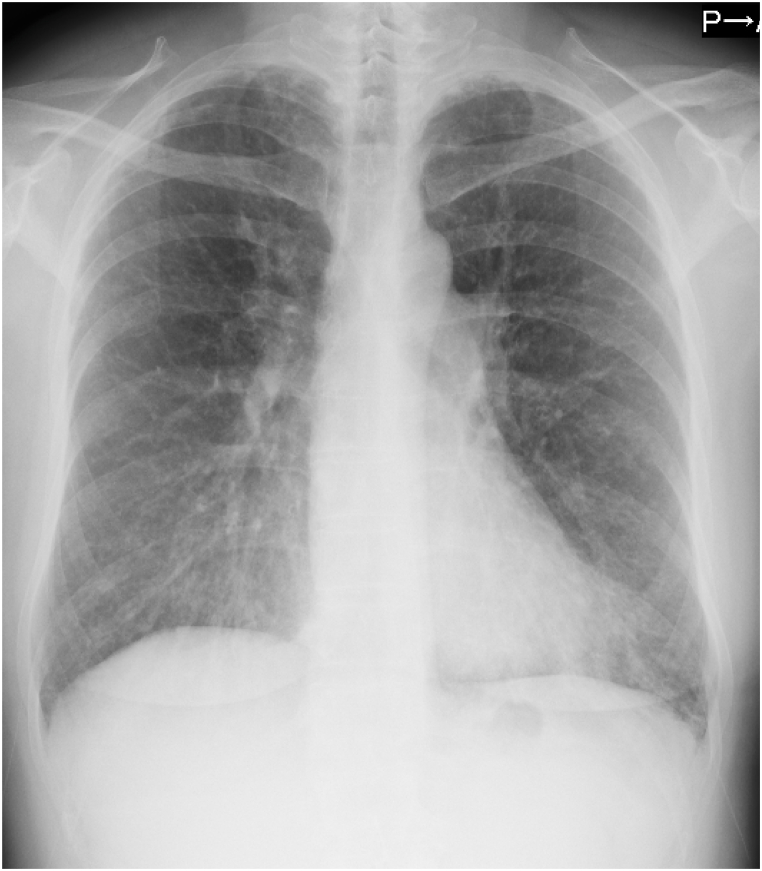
Chest X-ray showing diffuse granular shadows in both lungs.
